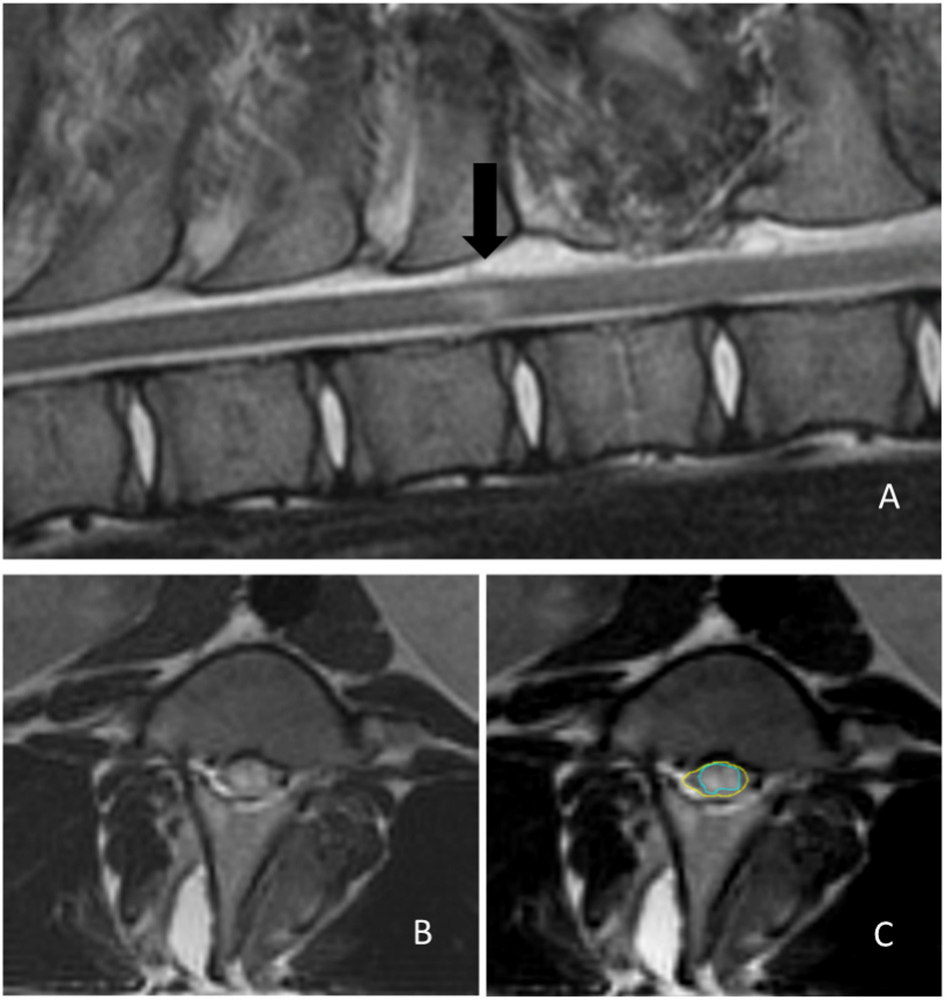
Determination of the extent of the trauma-induced region on magnetic resonance images. (A) Mid-sagittal T2-weighted image showing an intramedullary hyperintensity lesion (black arrow) indicating cord damage. (B) A segment of the maximal hyperintensity area in a transverse T2-weighted image. (C) The severity of the spinal cord injury is determined by comparing the hyperintense area (green circle) ratio to the total cross-sectional area of the spinal cord (yellow circle) using ImageJ software.
